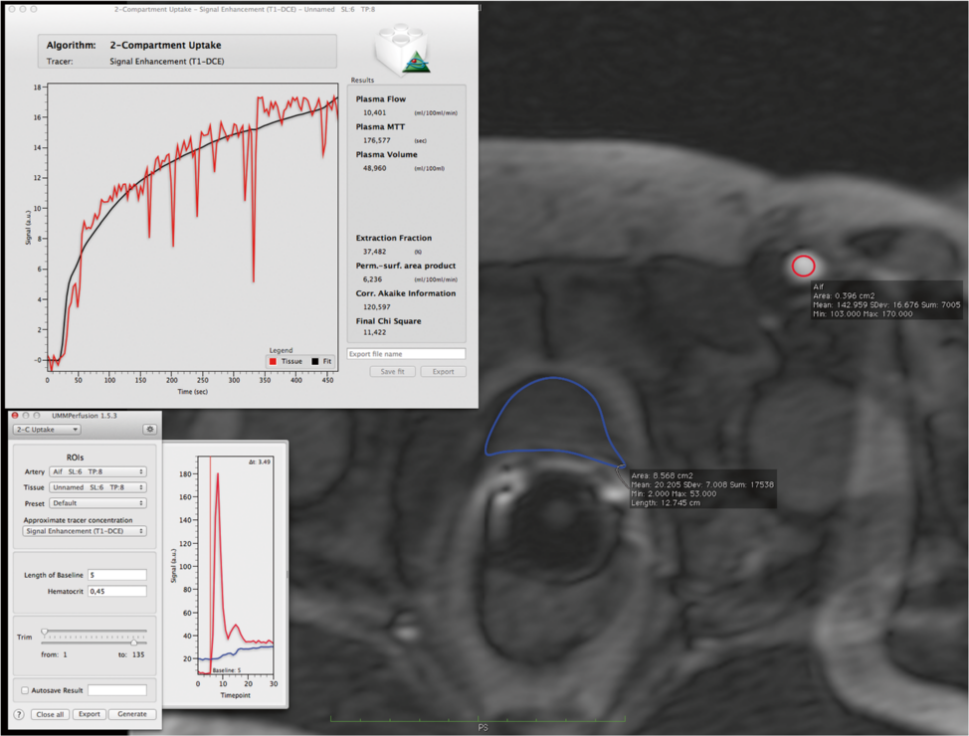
Example of ROI-based data analysis and result for a DCE-MRI of the prostate. The window in the top left area of the figure depicts the result of this analysis, showing the fit (*black curve*) to the data (*red curve*) and also listing the calculated parameters and GOF measures
